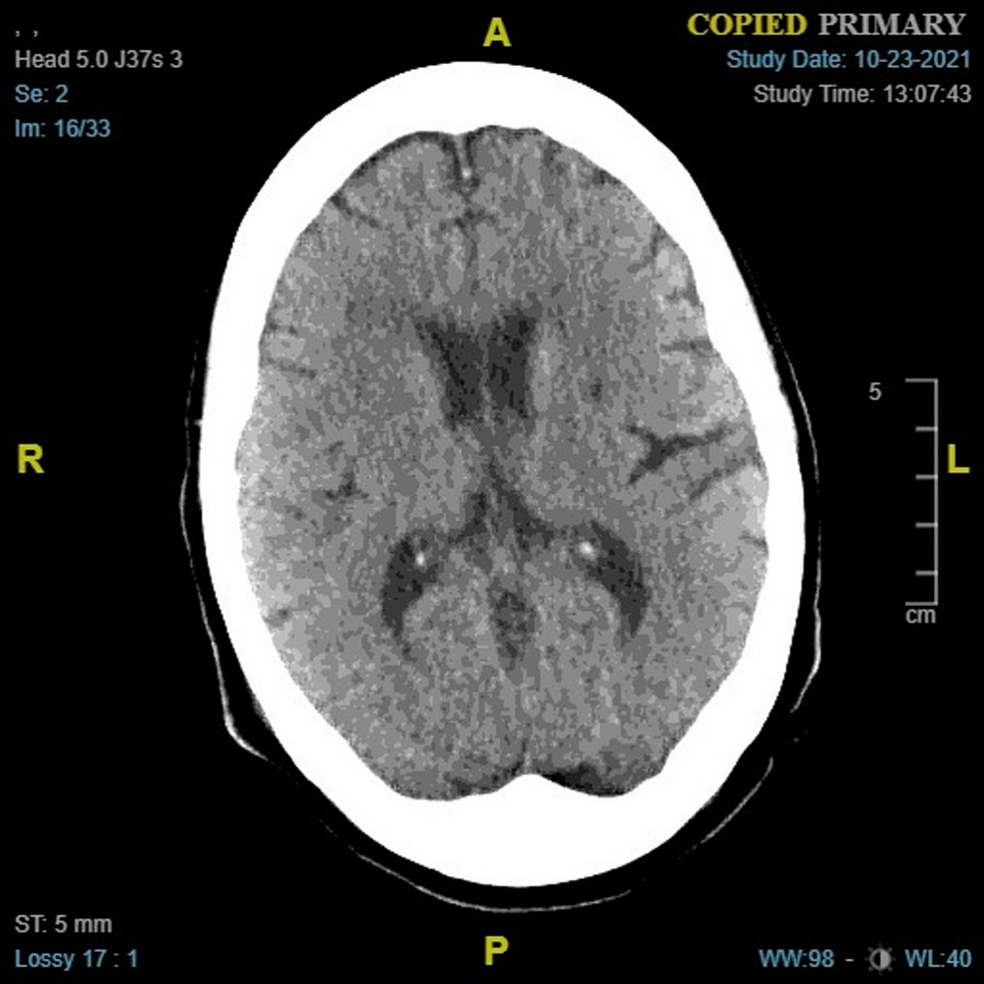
CT head of the patient with no acute intracranial process
